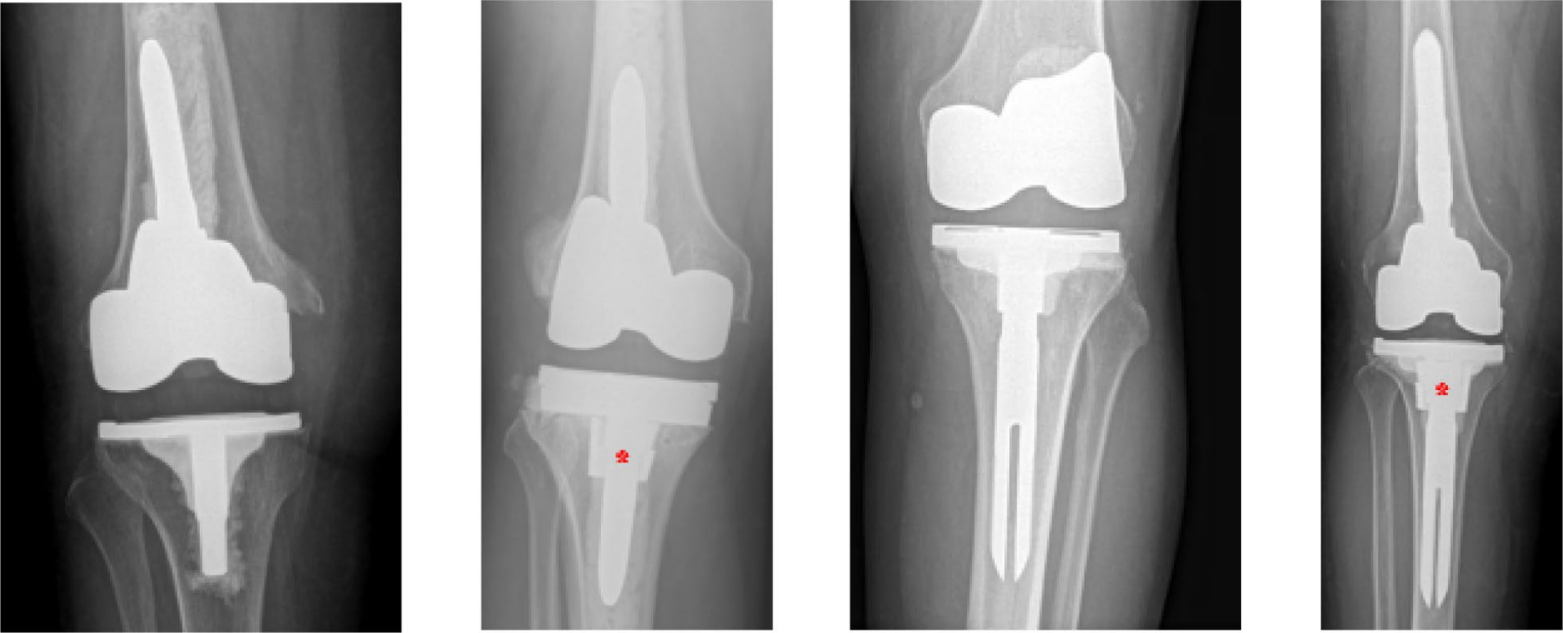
Radiographic imaging of cemented and hybrid stem technique, with and without cone augmentation. From left to right: cemented without cone; cemented with cone; hybrid without cone, hybrid with cone. *Indicates the presence of a cone
